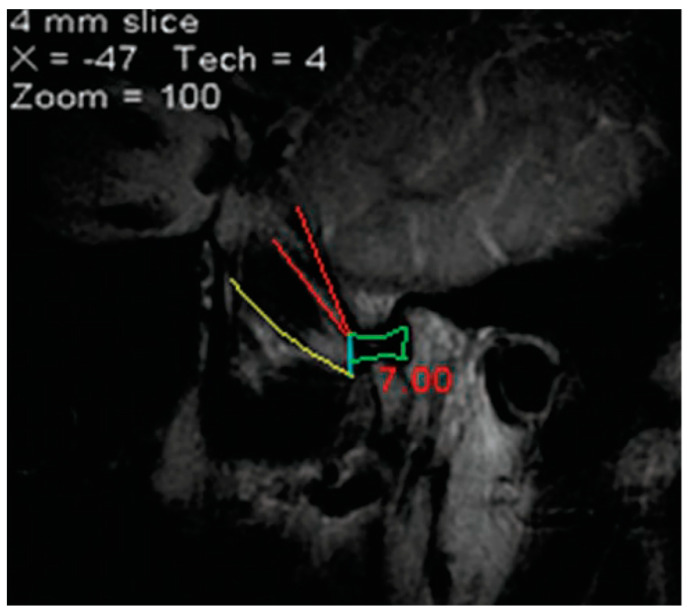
In the caption of Figure 1 in Pompei Filho’s paper [74], which was published under a CC BY-NC 4.0 license, the authors indicated: “MRI image of the TMJ, the superior and inferior margins of the third head of the lateral pterygoid muscle are bound with red. In yellow is observed in the inferior margin of the THLPM, with its insertion area into the disc in blue. The joint disc narrows in green.” THLPM is the abbreviation the authors used for the third head of the lateral pterygoid muscle [74]. The authors drew red lines on the margins of that THLPM, then they drew a lower yellow line to indicate, again, the inferior margin of the THLPM. The insertion area on the TMJ disc (blue line) is larger than the marked green area of the disc. We could not understand the meaning of the sentence “The joint disc narrows in green”. It was used as a measurement that is not explained in the caption of that figure. The osseous landmarks are not indicated and that MRI image was not oriented anatomically.
